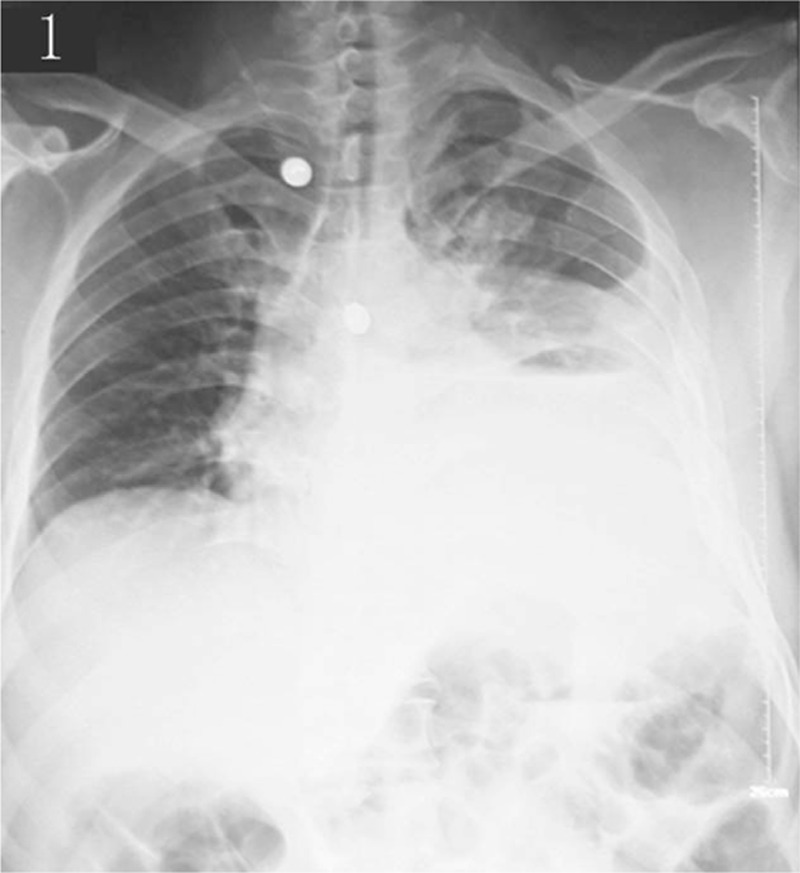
Chest x-ray revealing a left gastrothorax.
